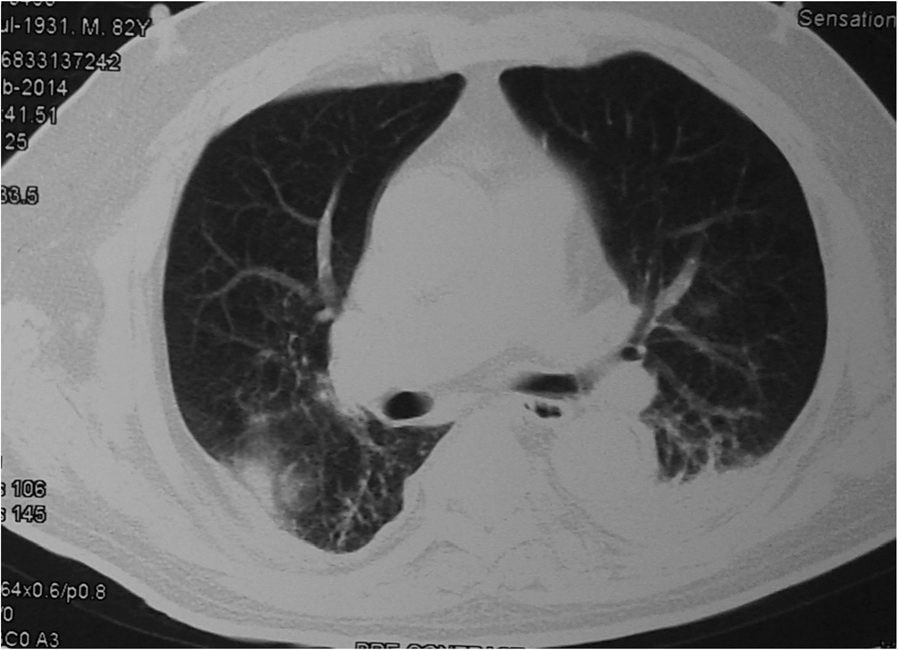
The patient (weight = 73 kg) was treated with intravenous methylprednisolone (80 mg/day for 8 consecutive days). Chest CT scan demonstrated decreased density and extent of pulmonary opacification involving both lungs
